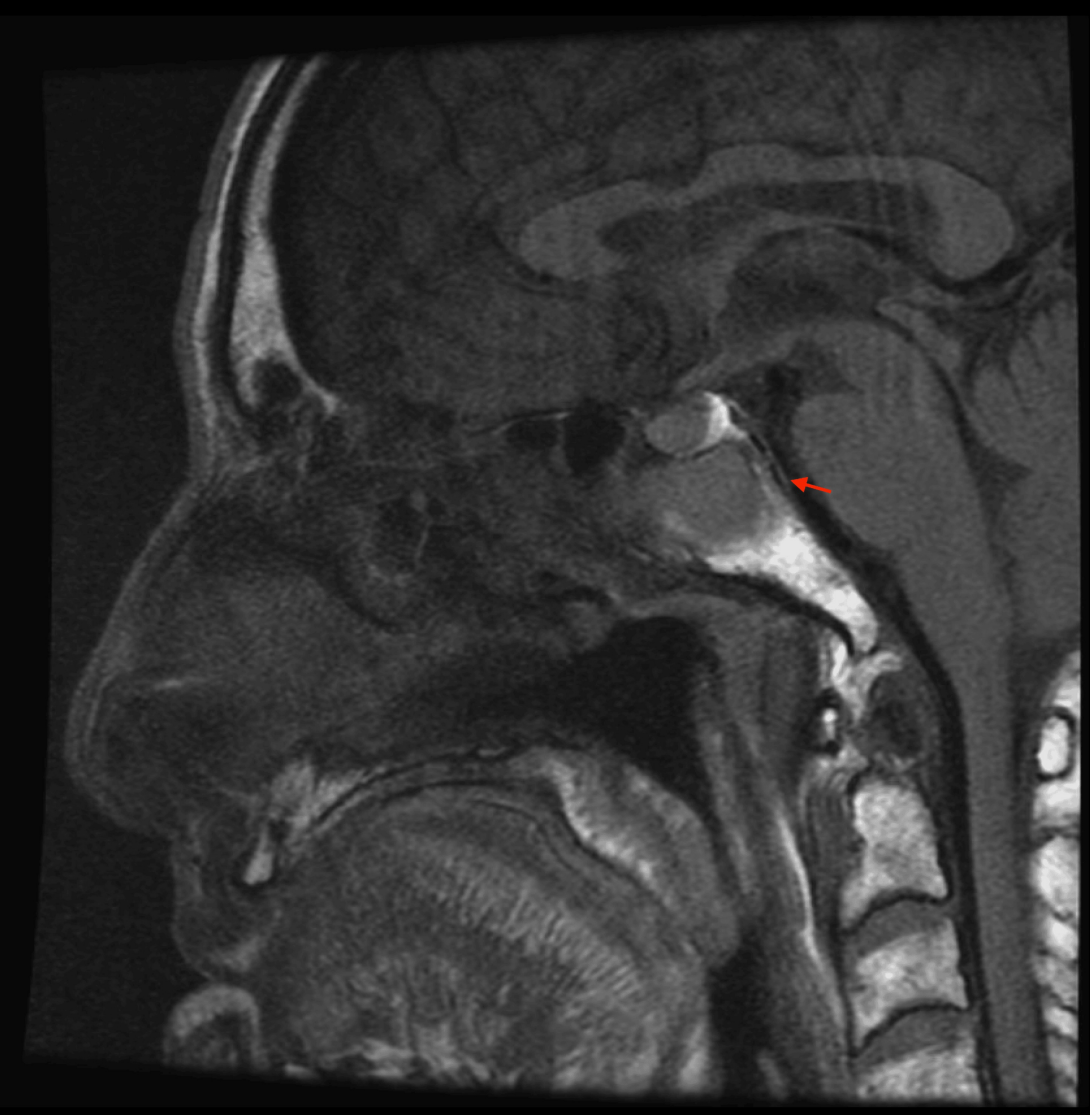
MRI with and without contrast of the orbit demonstrated abnormal T1 marrow suppression within the anterior superior margin of the clivus/sella turcica (arrow), concerning osteomyelitis, along with purulent debris filling the left sphenoid sinus and extruding through the sphenoid ostium (per radiologist's read) MRI: magnetic resonance imaging
